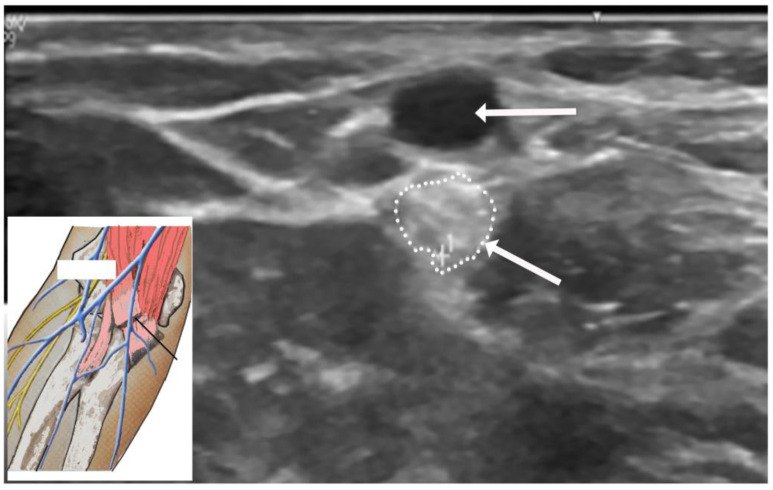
Ultrasound of injury to the lateral antebrachial cutaneous nerve from phlebotomy. Horizontal arrow points to the cephalic vein. Oblique arrow points to enlarged and hyperechoic lateral antebrachial cutaneous nerve. Oblique arrow points to the enlarged and hyperechoic lateral antebrachial cutaneous nerve (encircled by small dots).
